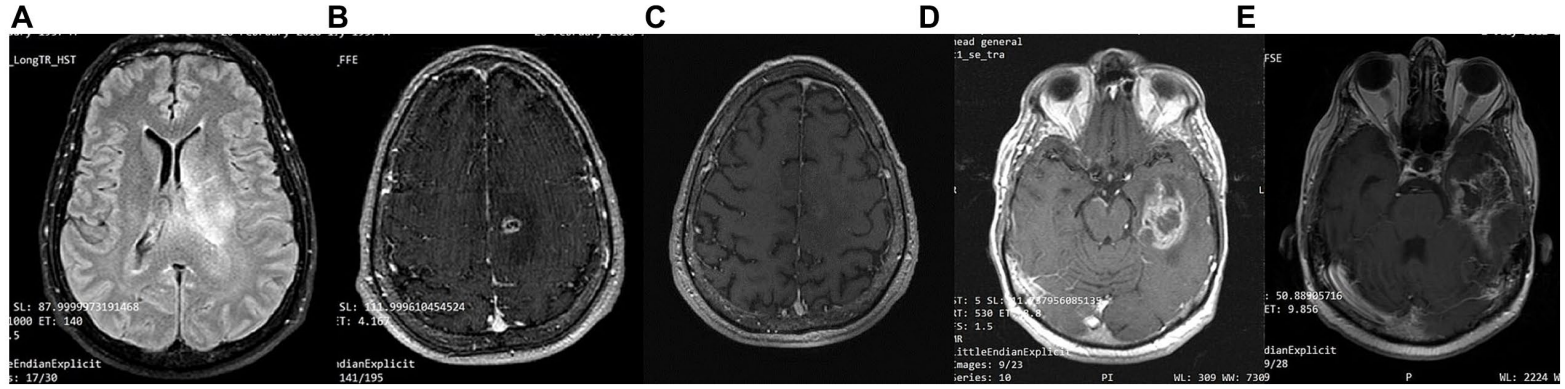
Patient 4: (A) brain MRI on diagnosis (T2/FLAIR) (B) brain MRI on diagnosis (T1 with contrast) (C) 24-month follow-up brain MRI (T1 with contrast) (D) 32-month follow up brain MRI. GBM relapse (T1 with contrast) (E) 41-month follow up brain MRI (T1 with contrast).
